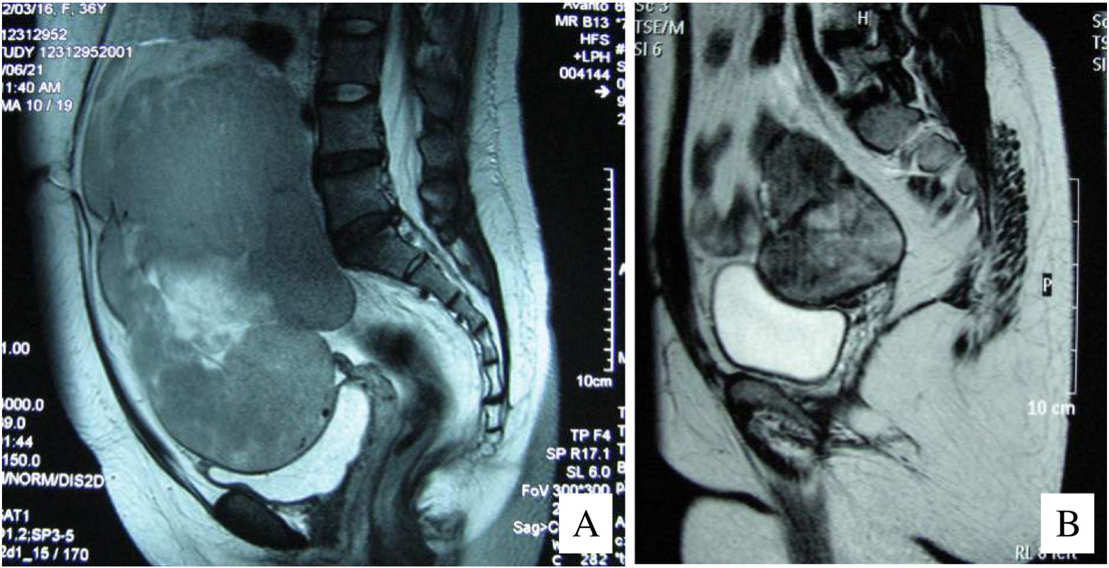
Magnetic resonance imaging of a tumor developing from the left testis in a patient with androgen insensitivity syndrome. (A) Before chemotherapy, a T2-weighted image showed that the internal intensity was slightly high. (B) Marked shrinkage of the tumor occurred after 3 cycles of neoadjuvant chemotherapy (NAC).
